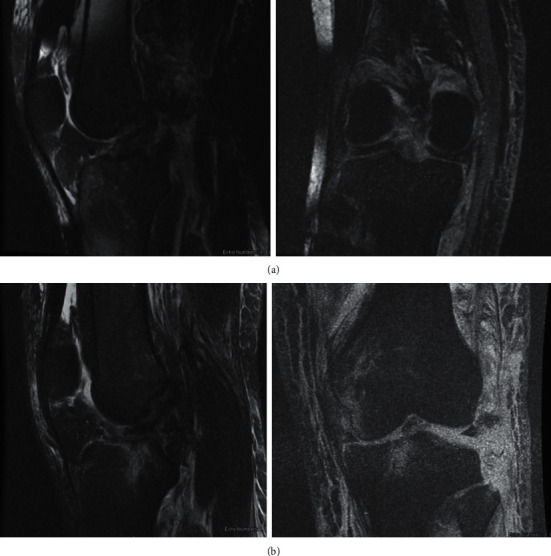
(a) MRI of the right knee depicting ACL and PCL rupture (left) and MCL rupture (right). (b) MRI of the left knee depicting ACL avulsion and PCL rupture (left) and LCL avulsion and PLC injury (right).
